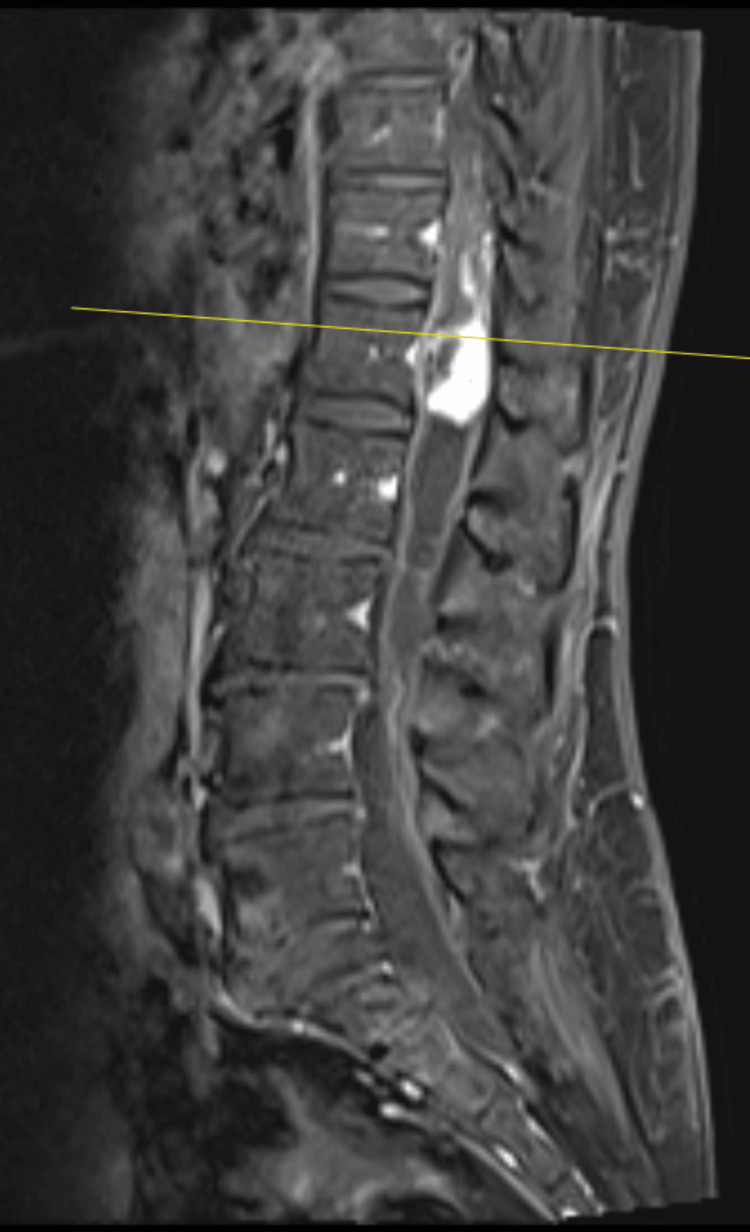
Pre-operative T2-weighted sagittal MRI image of intradural lesion at the level of T12.
